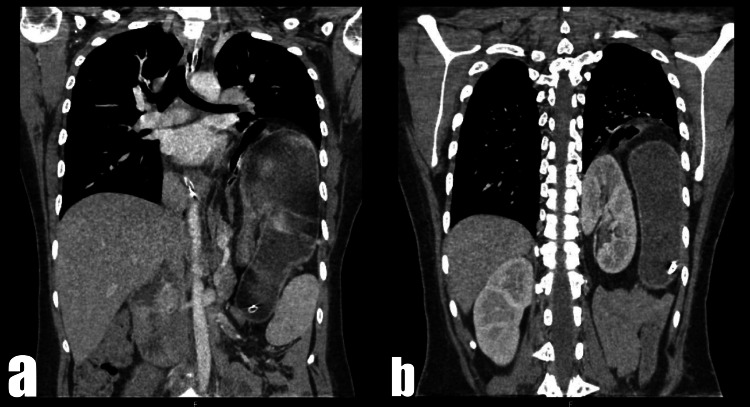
CT thorax and upper abdomen coronal sections showing (a) herniation of the stomach and (b) ectopic location of left kidney. CT, computed tomography
